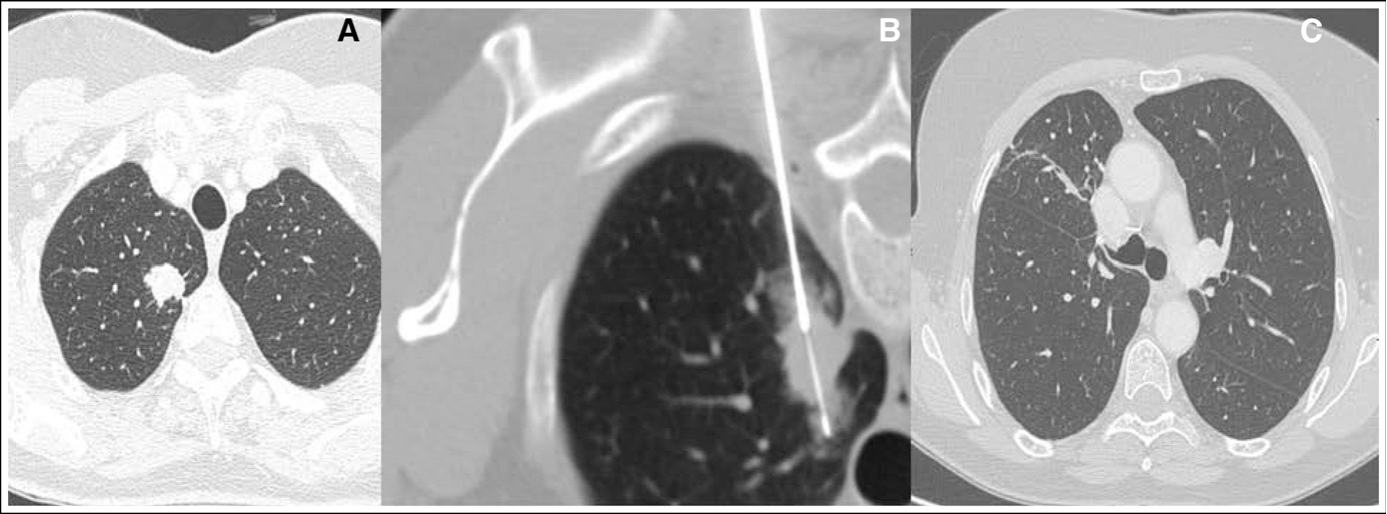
Computed tomography-guided pulmonary nodule biopsy performed on a patient included in the present study.
